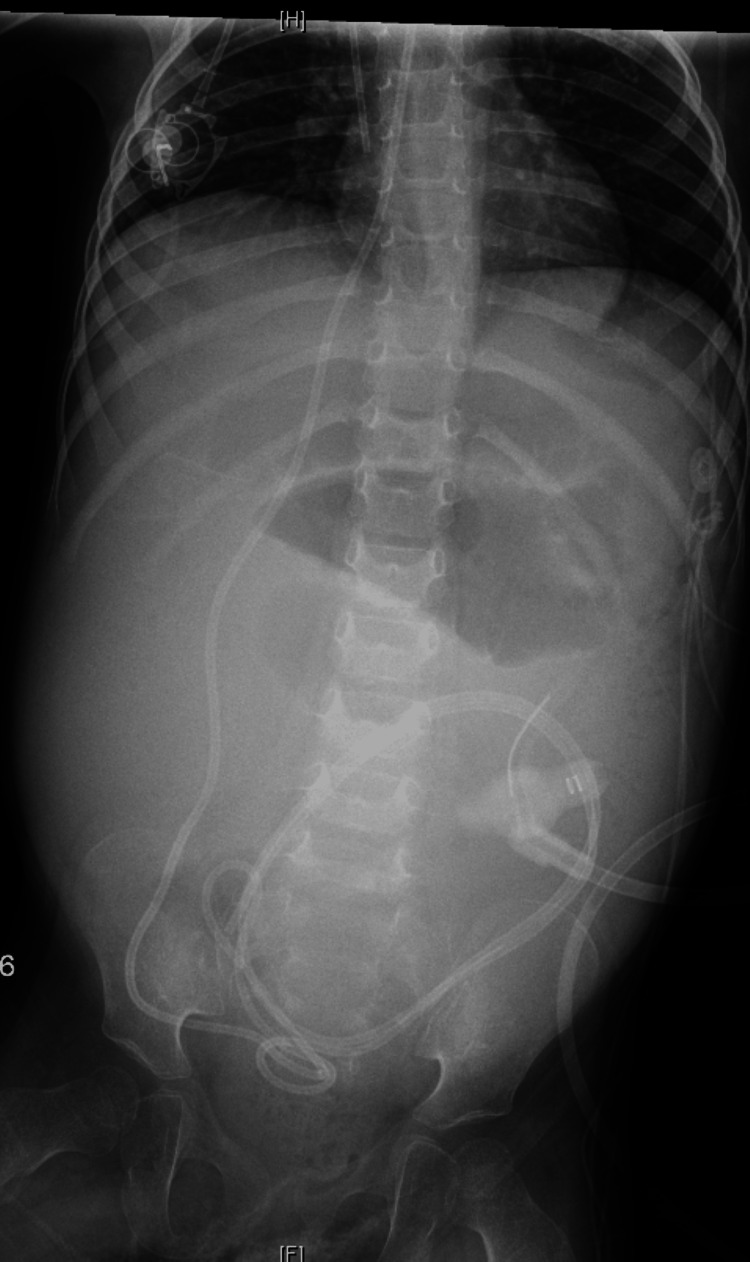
Initial abdominal X-ray revealing nonspecific bowel gas pattern with aerated loops of bowel most consistent with intestinal ileus
